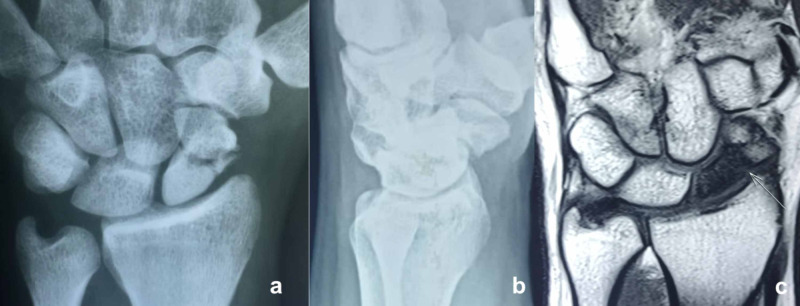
Posteroanterior (a) and lateral radiographs (b) of a 29-year-old man with left side scaphoid nonunion and avascular necrosis of 36 months duration. MRI (c) showing scaphoid nonunion with a T1-weighted coronal image showing diffusely decreased signal within the proximal pole We identified no bleeding at the surgery.
